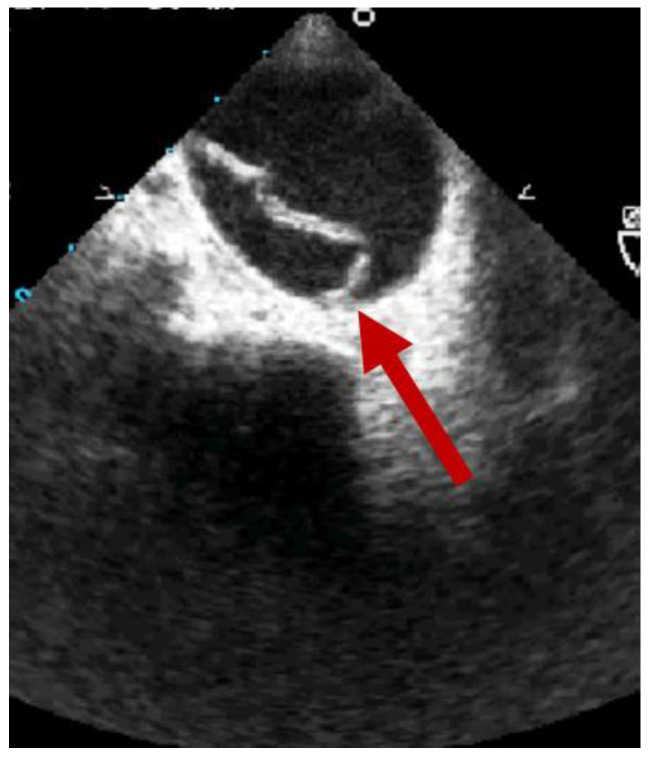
Transesophageal echocardiography showing the presence of an intimal flap (red arrow).
